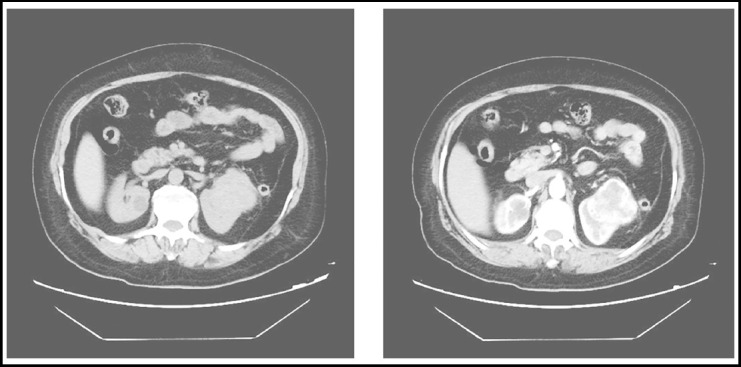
CT Image of Renal Tumor. (A: Irregular density shadow was seen in the left kidney on plain scan; B: There was obvious inhomogeneous enhancement in arterial phase in contrast-enhanced scan.).
